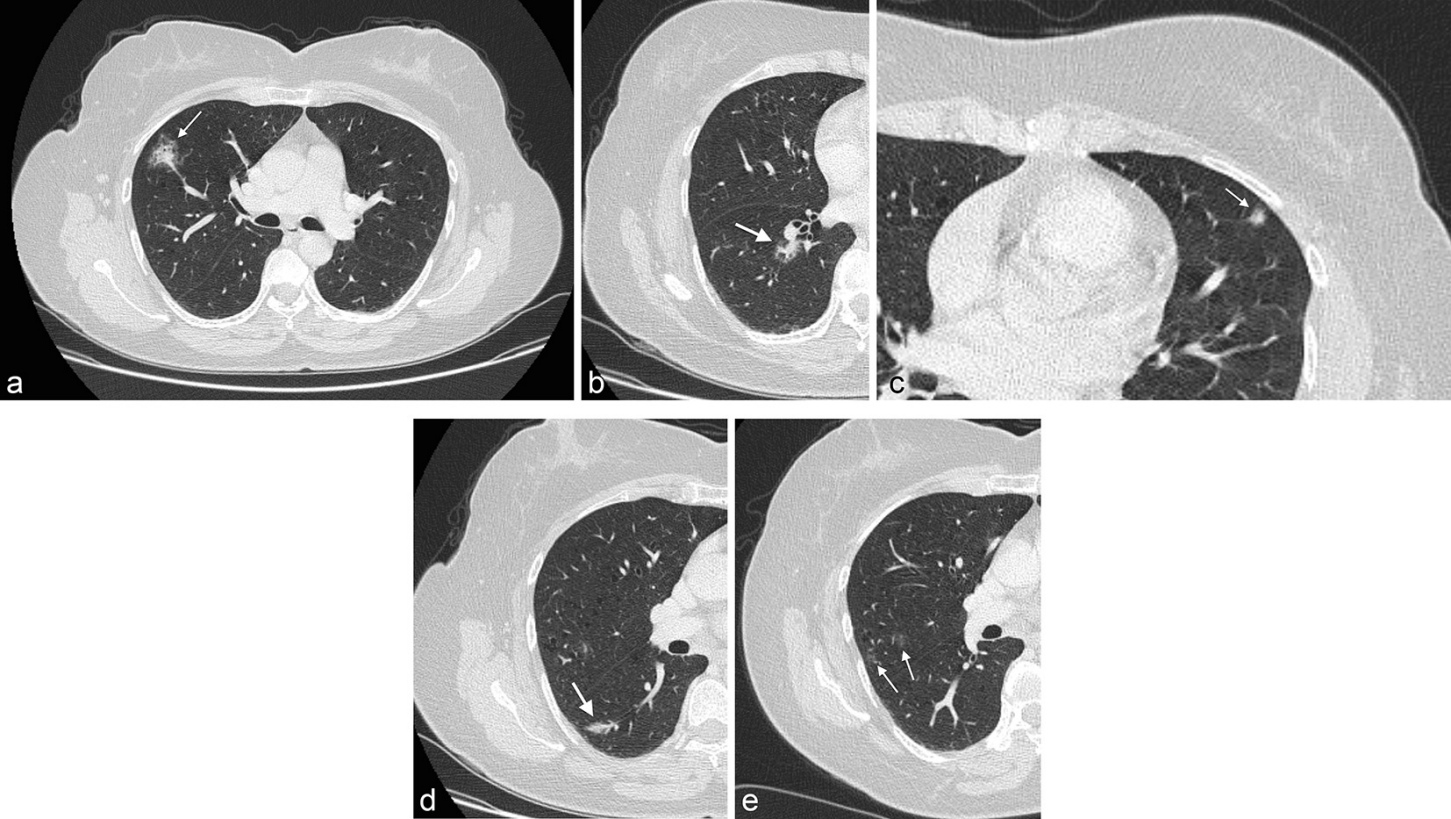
(a–c) CT images showing part-solid opacities with central solid component surrounded by a ground-glass opacity halo. (d) Sub-solid non-round ground-glass opacity. (e) Example of two ill-defined pure ground glass opacities.
